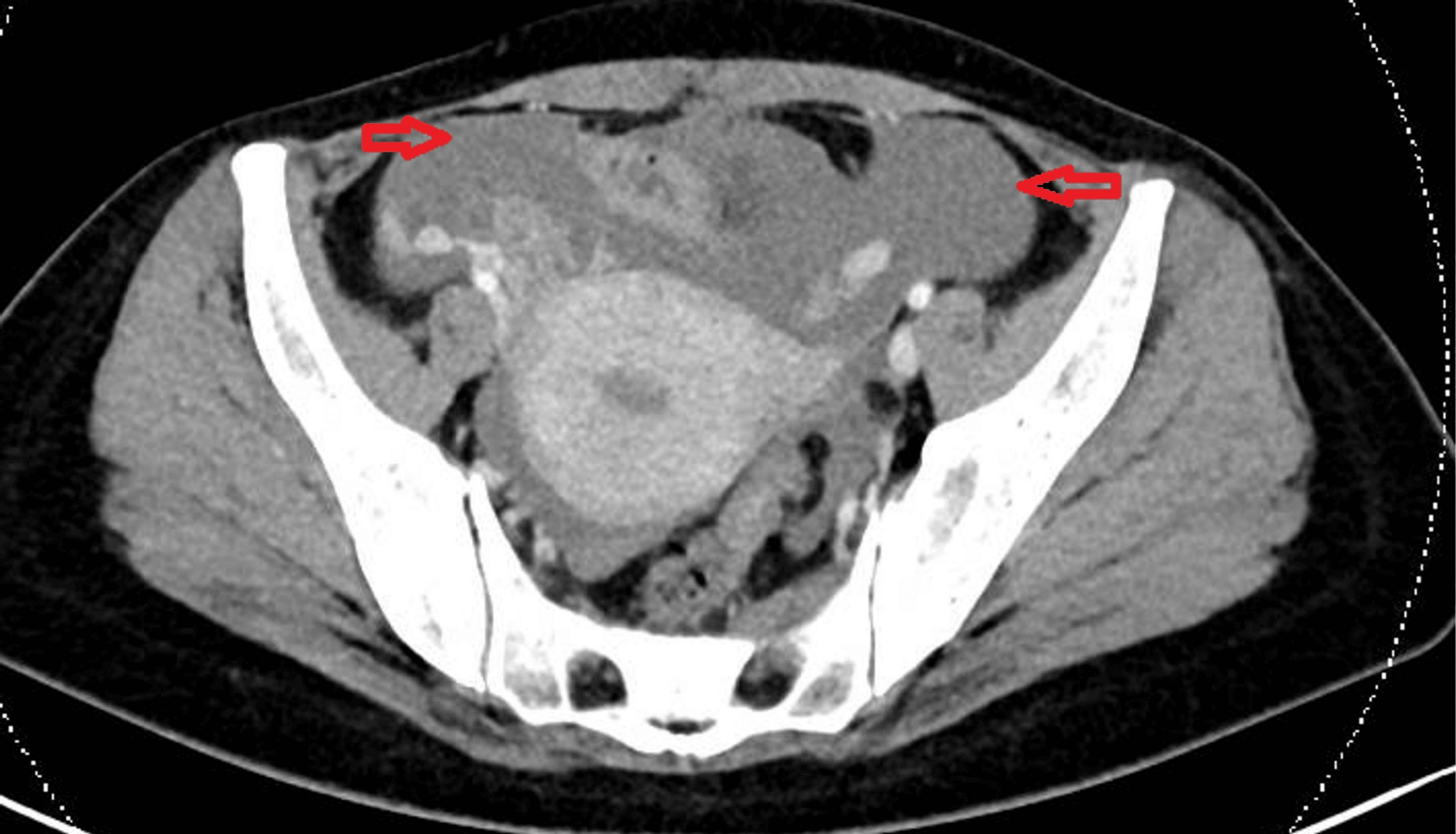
CT abdomen and pelvis of the patient showing large amount of hemoperitoneum (red arrows). The CT scan was performed at around two hours after the initial presentation.
